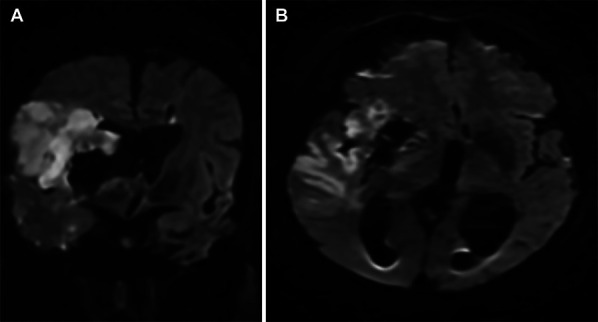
Postoperative MR imagesafter endoscopic hematoma evacuation. Both the A, coronal and B, axial diffusion weight MR scans show an infarct area in the middle cerebral artery region during the postoperative period. MR, magnetic resonance.
